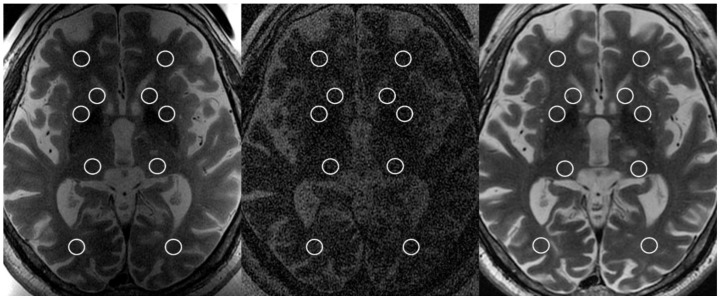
Axial T2-weighted FRFSE images acquired intraoperatively using the dedicated two-channel FUS-Head coil (left image); body radiofrequency coil (central image); and at 48-h using the eight-channel phased-array head coil (right image) at the level of the anterior commissure-posterior commissure plane, showing the placement of regions of interest for the evaluation of signal-to-noise ratio.
